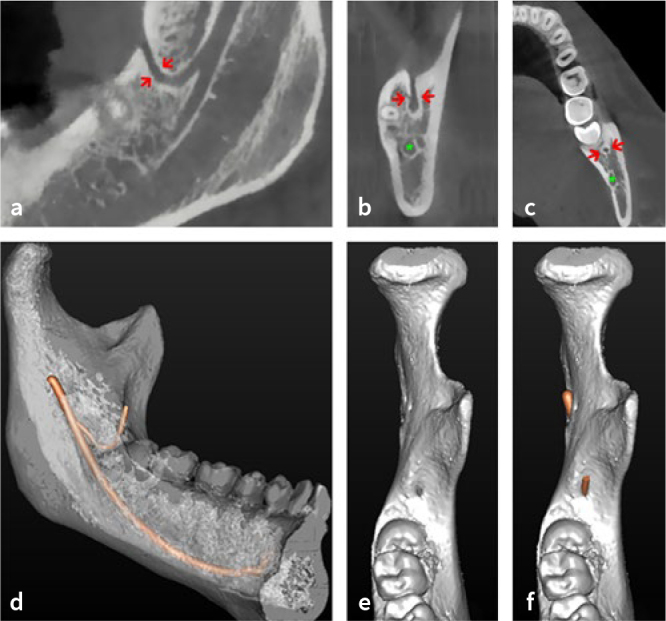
CBCT images of a retromolar canal: a. Sagittal CBCT slice showing a vertical retromolar canal (red arrows) from the bifurcation point to the retromolar foramen; b. coronal CBCT slice showing the last section of the retromolar canal (red arrows) and its opening (retromolar foramen) and the mandibular canal (green asterisk); c. axial CBCT slice showing a section of retromolar canal (red arrows) and a section of the mandibular canal (green asterisk); d. volumetric reconstruction with a transparency kernel that highlights the entire course of the retromolar canal; e. detail of the volumetric reconstruction showing the retromolar foramen; f. detail of the volumetric reconstruction showing the vascular-nerve bundle that crosses the retromolar foramen.
